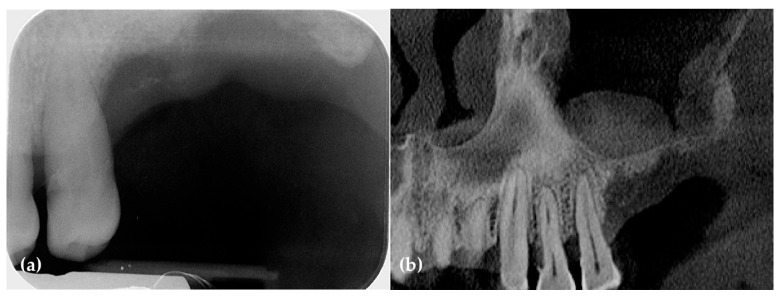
(a) Severe vertical bone defects are observed in the preoperative periapical radiograph; (b) The preoperative panoramic CBCT scan images also reveal significant vertical bone defects.
